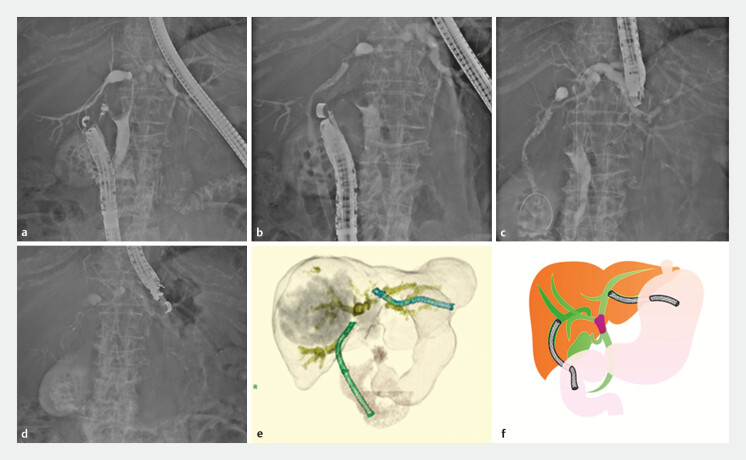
A case of endoscopic ultrasound-guided hepaticoduodenostomy and hepaticogastrostomy (EUS-HDGS) with a metallic stent (MS).
